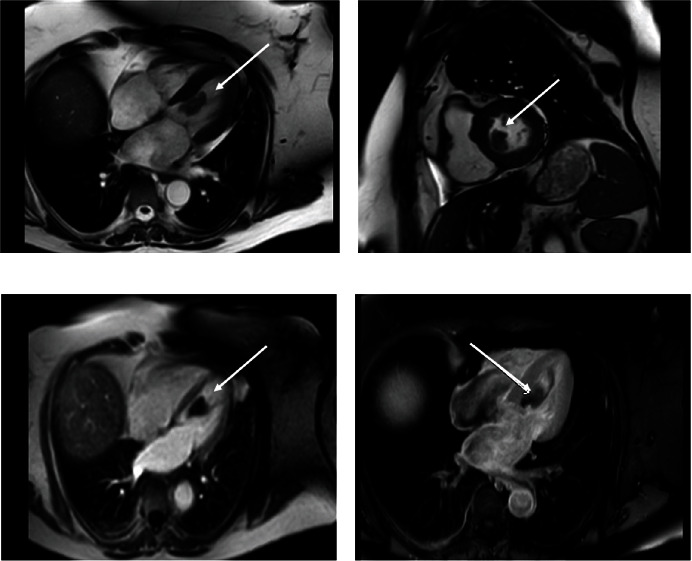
Cardiac MRI. Panels (a), (b), (c), and (d) show in different sequences a mass attached to the mitral valve apparatus and interventricular septum.
